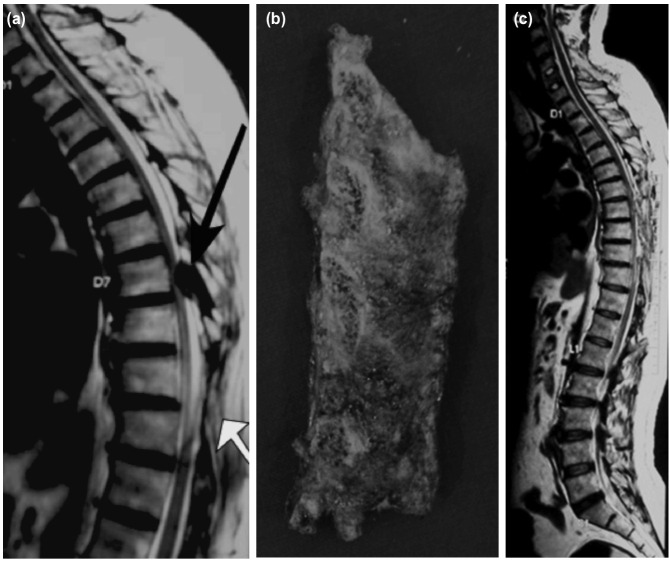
At 2-year follow-up of a patient, operated for T9-10 stenosis with conventional method developed a new stenosis at T7-8. The 1st ORT was 90 minutes and a post-operative deficit occurred. It was complete paraplegia and then recovered slowly beyond a pre-operative mJOA of 5 to final mJOA of 9 at 12 months. At the 2nd presentation of the same patient, the mJOA score again deteriorated to 6. Second surgery was with UBS and with a quick ORT of 55 minutes. There was no post-operative deficit in second surgery, mJOA again improved to 9 and maintained at 5-year follow-up. (a) T2 sagittal MRI (white arrow showing operated laminectomy and black arrow showing new stenosis). (b) En-block laminectomy bone removed with bone scalpel (c) Post-operative T2 sagittal MRI showing adequate decompression.
